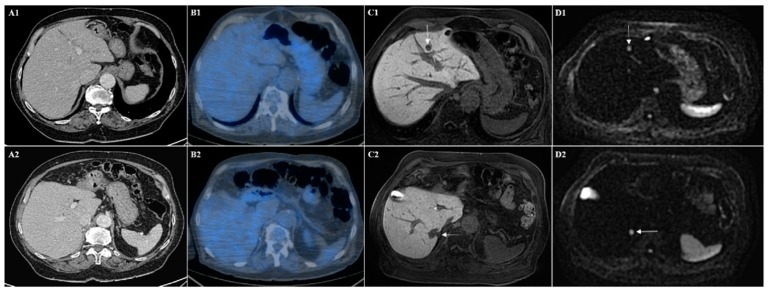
(A) CT and (B) PET-CT images show the absence of liver metastases in different liver section (1 and 2). (C) Hepatobiliary phase of Gd-EOB-DTPA MRI and (D) diffusion-weighted imaging show in the same patient the presence of two liver metastases from sarcoma in two different levels, one in the segment III (arrows in (C1) and (D1)) and one in the segment VII (arrows in (C2) and (D2)).
